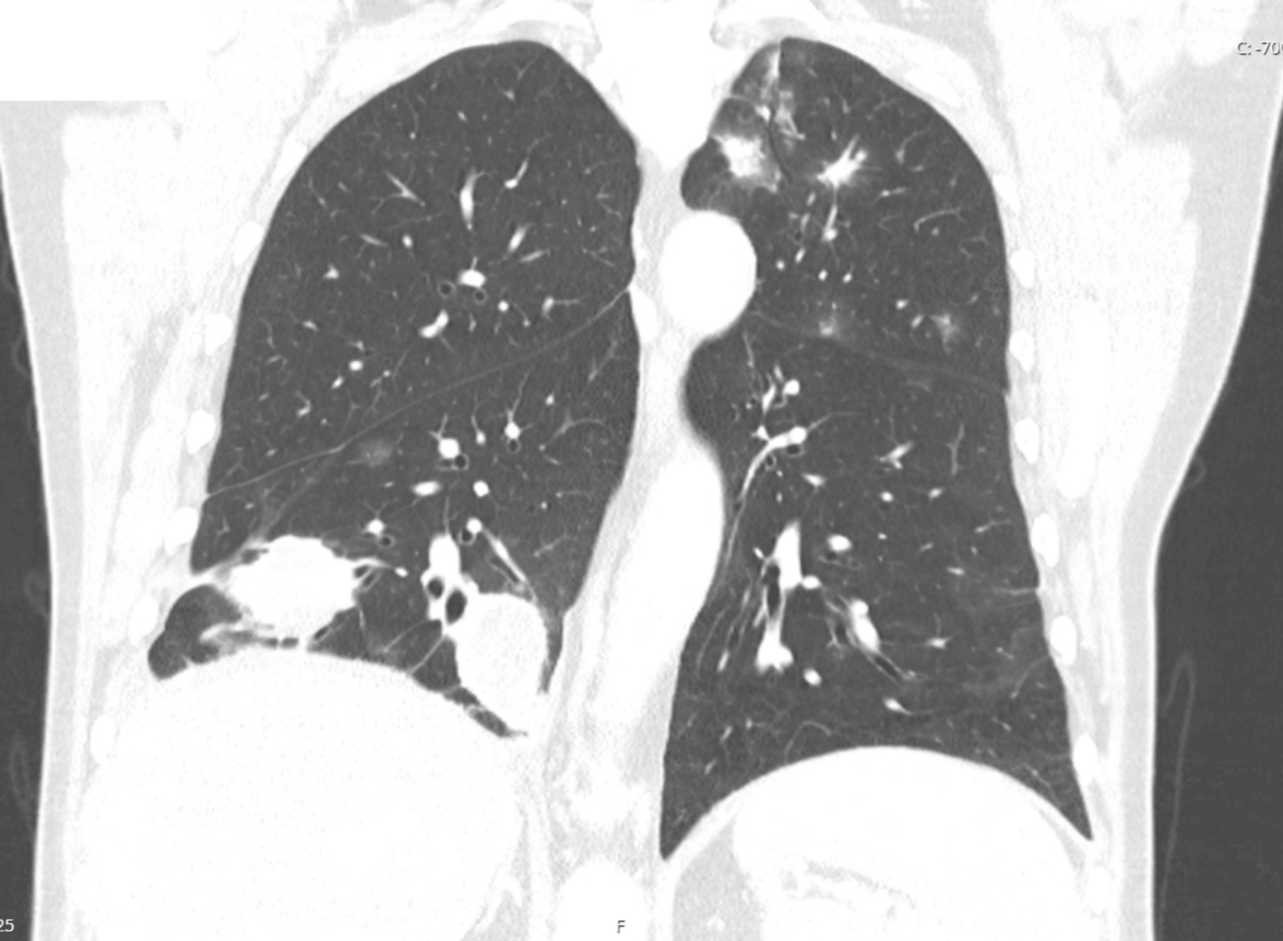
Chest CT (nine-month follow-up after immunotherapy initiation) Lung window, coronal view. Progression of pulmonary metastatic disease, with an increase in the size and number of bilateral pulmonary nodules: the largest lesions measure approximately 40 mm in maximum axial diameter in the right lower lobe (previously 23 mm), 21 mm in the left lower lobe (previously 16 mm), and 16 mm in the lingula (previously 14 mm); multiple new lesions are also observed, particularly in the upper lobes, the largest measuring 13 mm in the left upper lobe. CT: computed tomography
